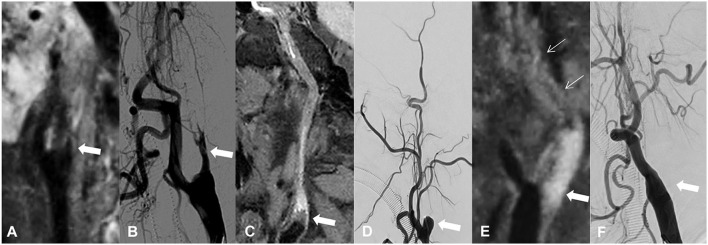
Classification of proximal stump in patients with CICAO. Arrows show the proximal stump condition on HR-MRI and DSA: (A,B) occlusion with tapered stump, (C,D) occlusion with blunt stump, and (E,F) occlusion with no stump.
